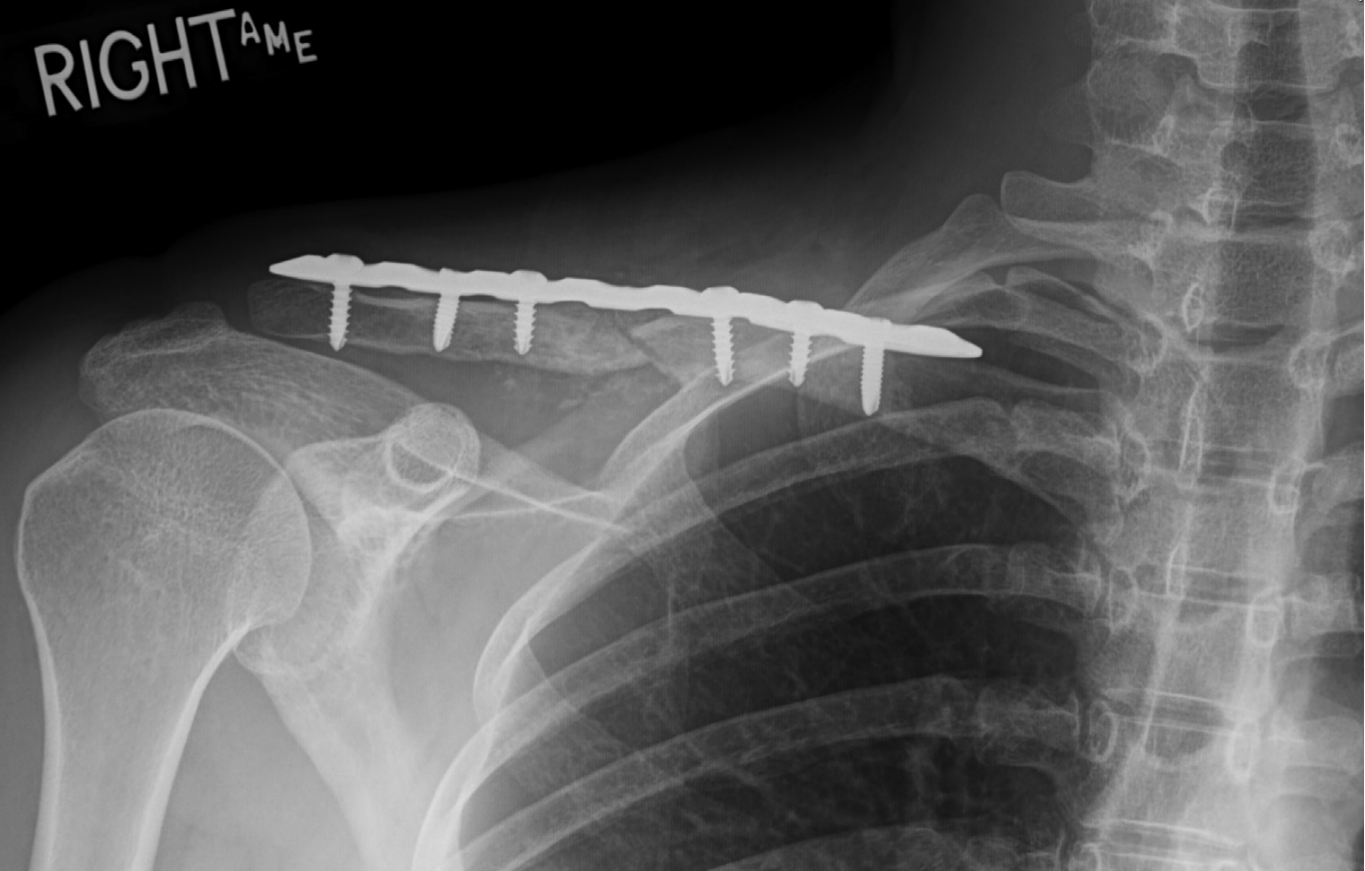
Postoperative anterior-posterior uptilt radiograph of *Right* clavicle.
